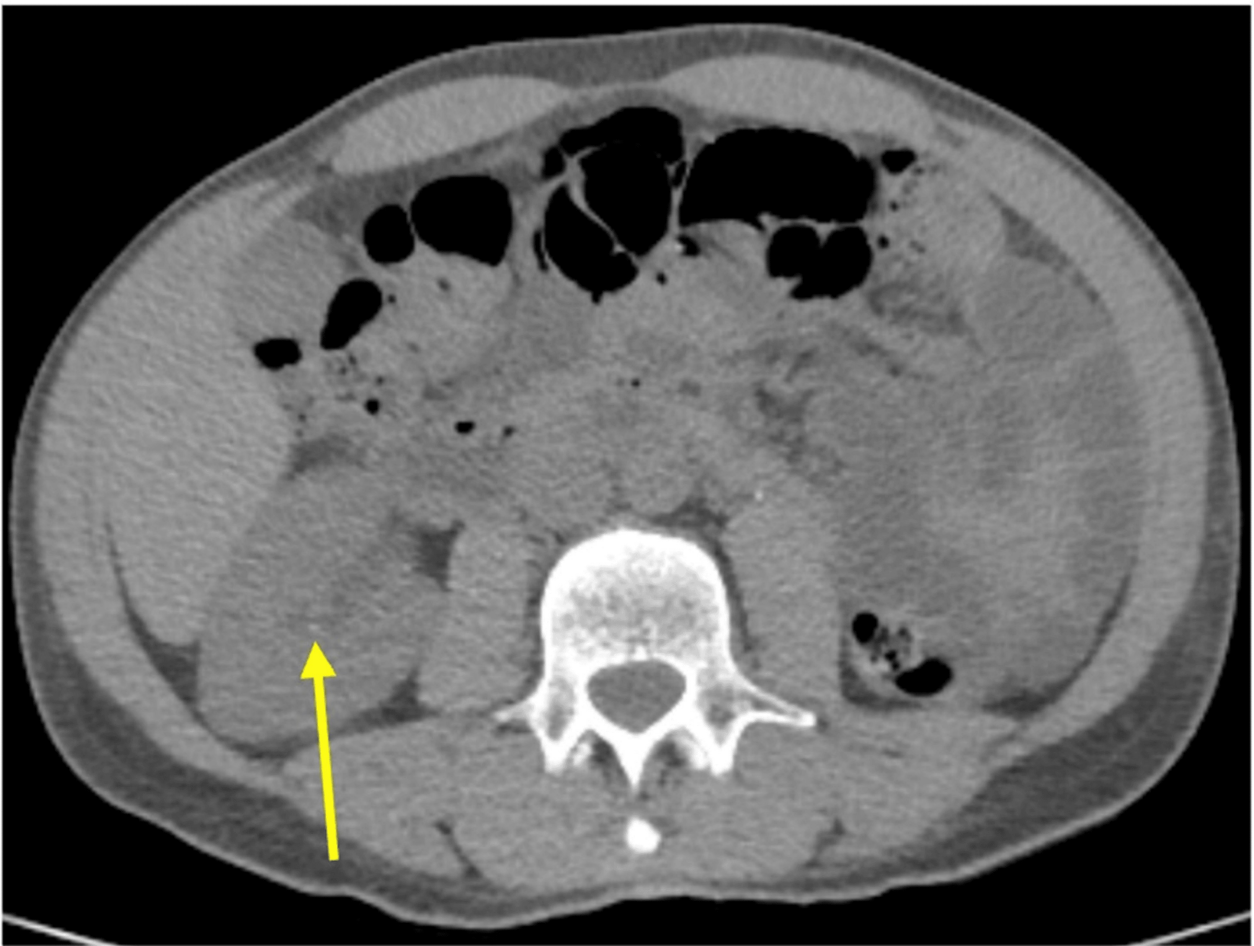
Non-contrast CT of the abdomen and pelvis showing a punctate calculus in the right interpolar calyx (yellow arrow) No associated hydronephrosis is observed in the solitary right kidney.
